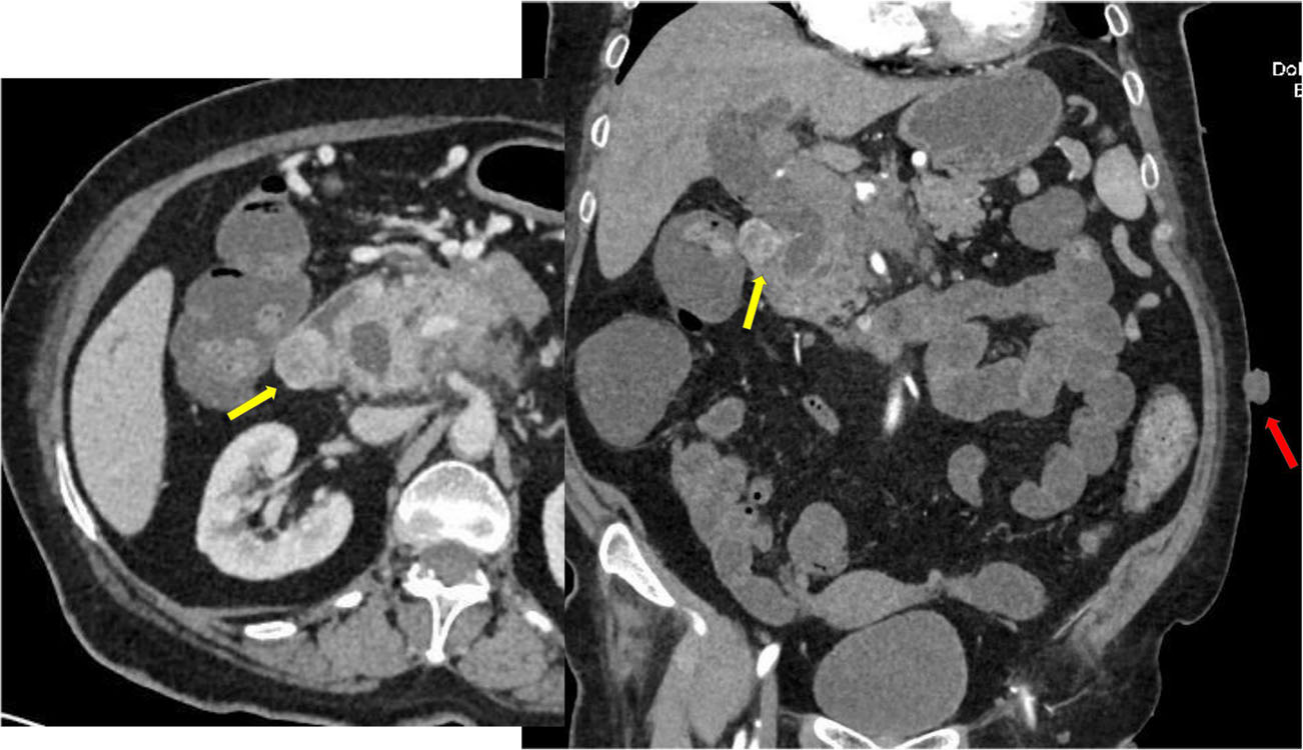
A 55-year-old female with systemic NF1 patient presenting a cholangitis. Dual-phase contrast-enhanced CT scan reveals a parietal pariampullary duodenal nodule highly enhanced by contrast (yellow arrows). Neurofibromas of the abdominal wall are also found on CT scan (red arrow)
